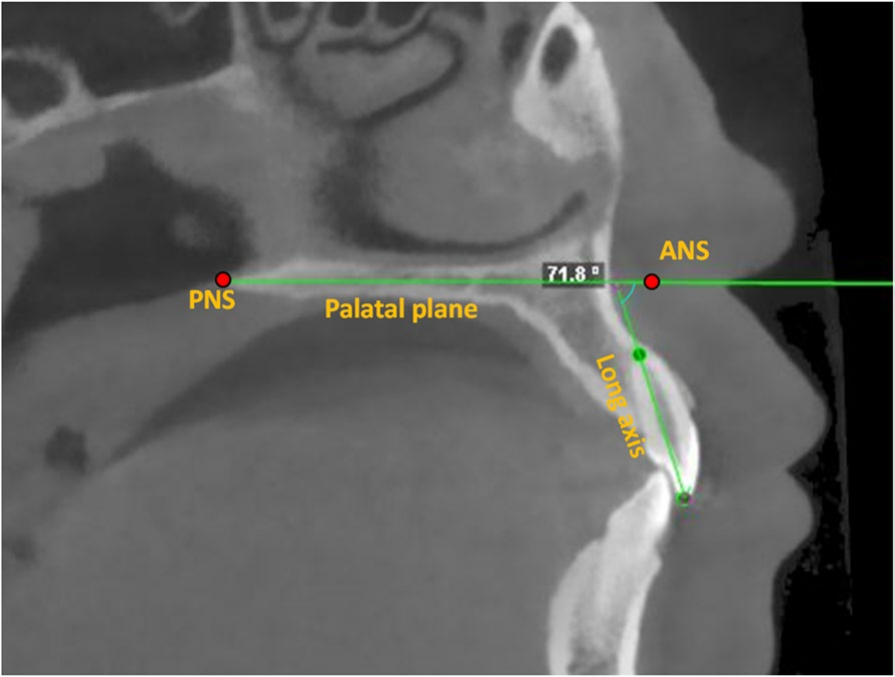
Measurement of bucco-palatal inclination of lateral incisors in CBCT. Bucco-palatal inclination of the maxillary lateral incisors were measured by the angle formed by the long axis of the maxillary lateral incisors and the palatal plane (ANS-PNS) in the sagittal plane. The larger the angle, the more labial inclination the root of the lateral incisor was
